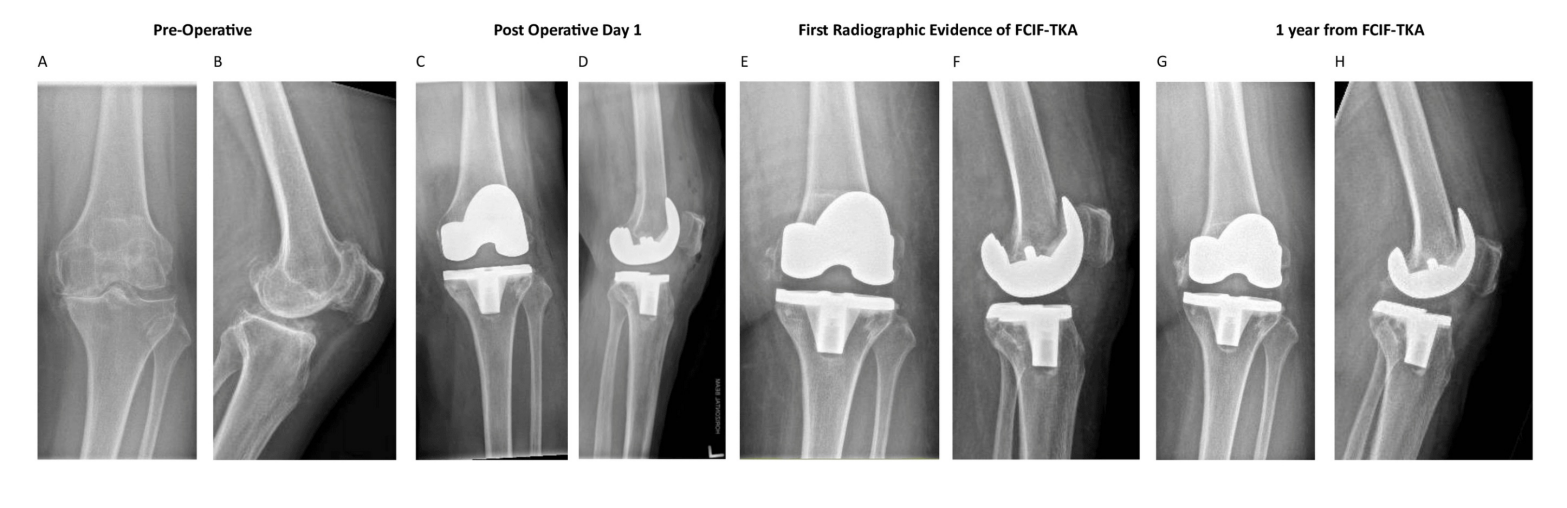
Patient 1 AP and lateral knee FCIF-TKA radiographs A: preoperative AP film, B: preoperative lateral film, C: postoperative day 1 AP film, D: postoperative day 1 lateral film, E: first radiographic evidence of FCIF-TKA AP film, F: first radiographic evidence of FCIF-TKA lateral film, G: one year from FCIF-TKA AP film, H: one year from FCIF-TKA lateral film AP: anterior-posterior, FCIF-TKA: femoral condyle insufficiency fractures following total knee arthroplasty
